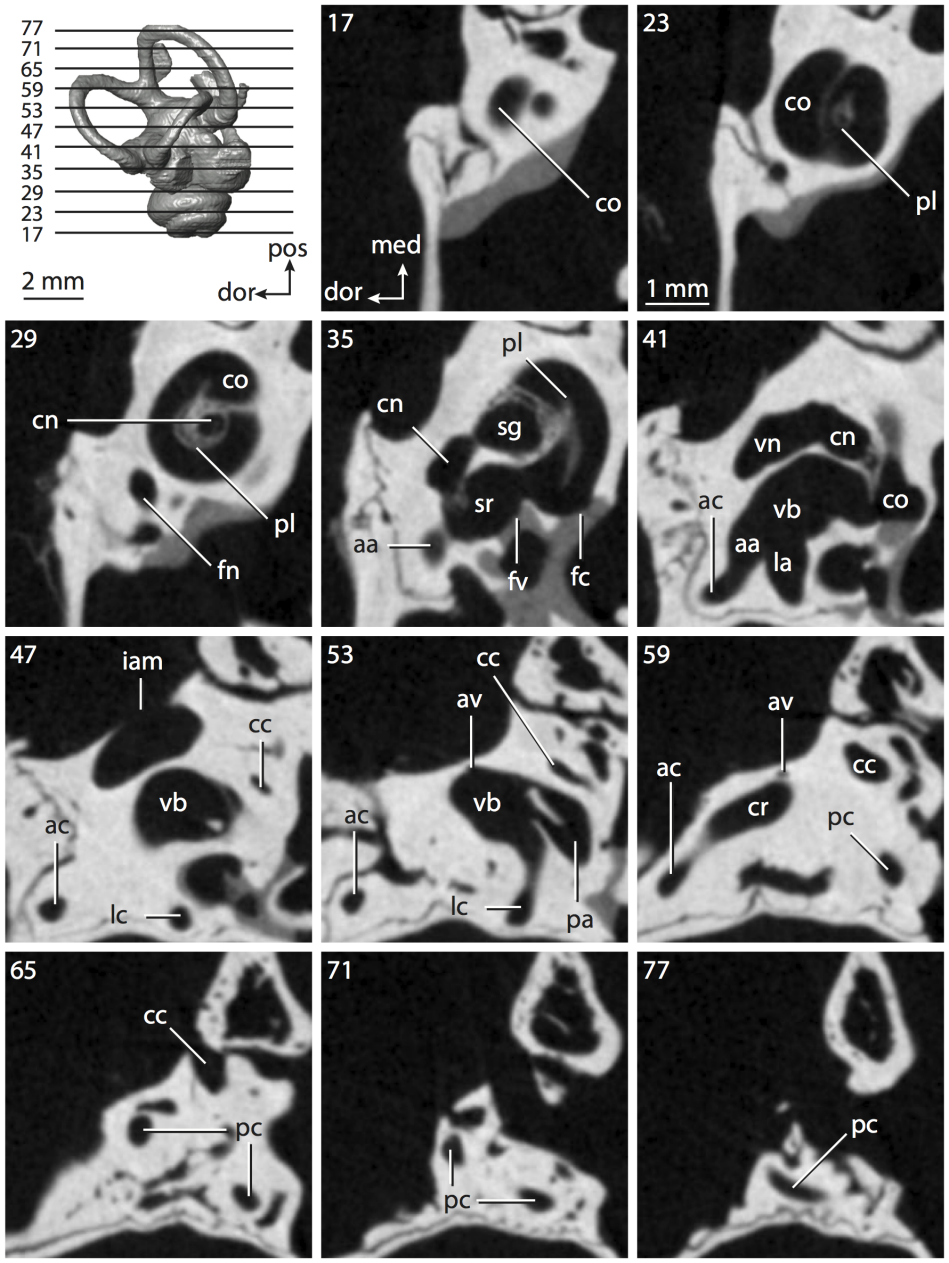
CT slices through ear region of*Manis tricuspis*. Abbreviations listed at the end of the Materials and Methods section.
